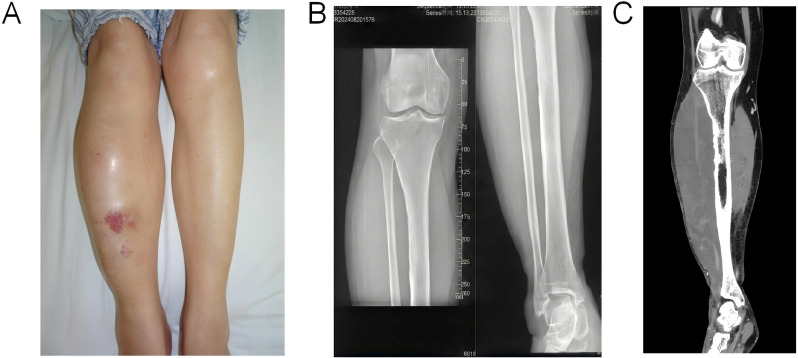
(A) Obvious swelling of the middle and lower right calf with local rash, lobulated nodules, and skin damage. (B) Anteroposterior (AP) and lateral X-ray images of the right tibia, showing an internal mass of the right tibia anterior muscle. (C) CT images of the right lower limb showing an extensive soft tissue tumor and tibial cortex involvement.
